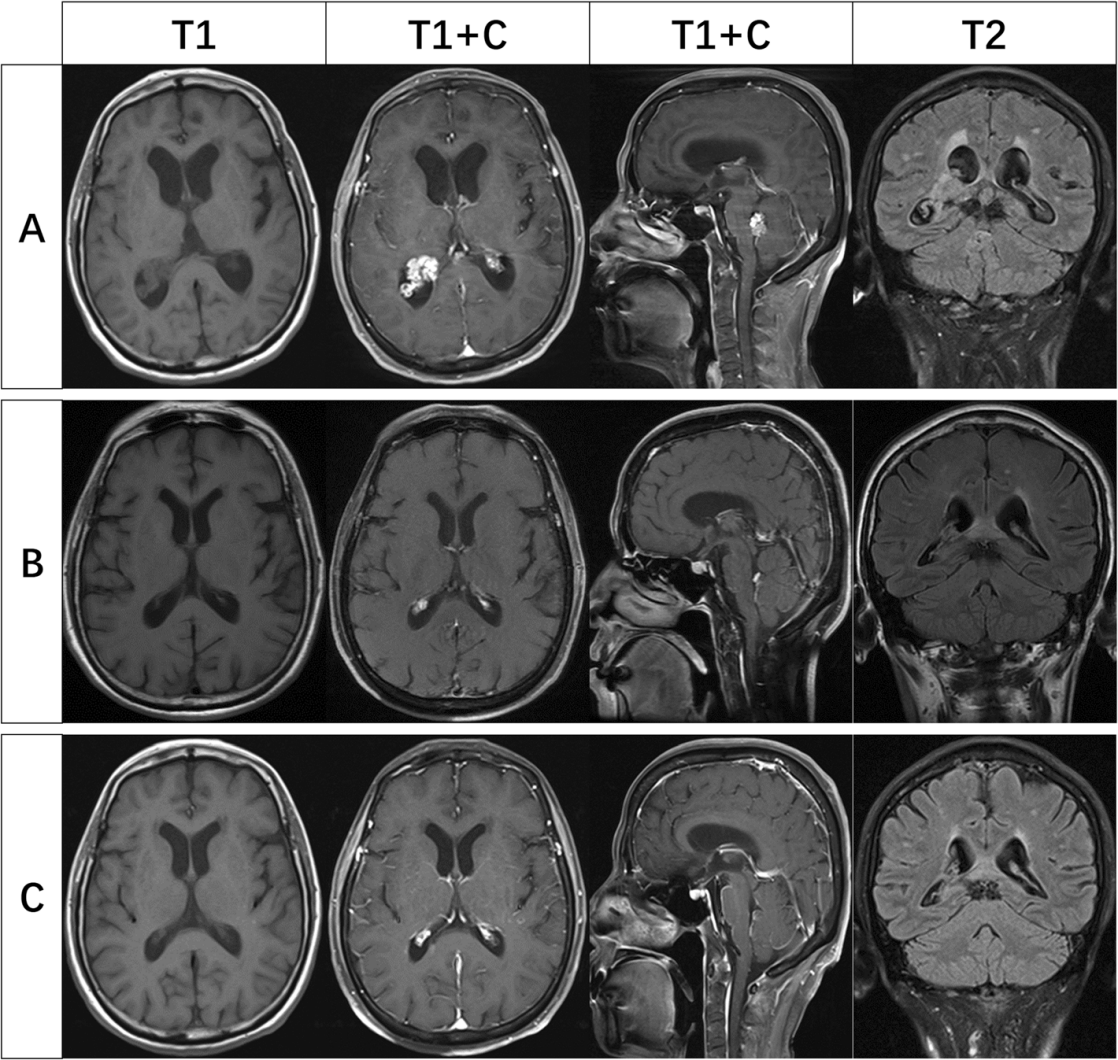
MRI of brain during different periods of treatment a Contrast-enhanced MRI of brain showed heterogeneously enhancing lesions at the posterior horn of the right lateral ventricle and the IV ventricle, with upper ventricle hydrocephalus and edema of the surrounding parenchyma. T2FLAIR showed slightly higher signal of the masses. b Intraventricular lesions and surrounding area of edema observably shrunk with restored ventricular dilatation after 3-month treatment with afitinib. c Masses in the brain remain stable after 10-month treatment while lesions of lung progressed
